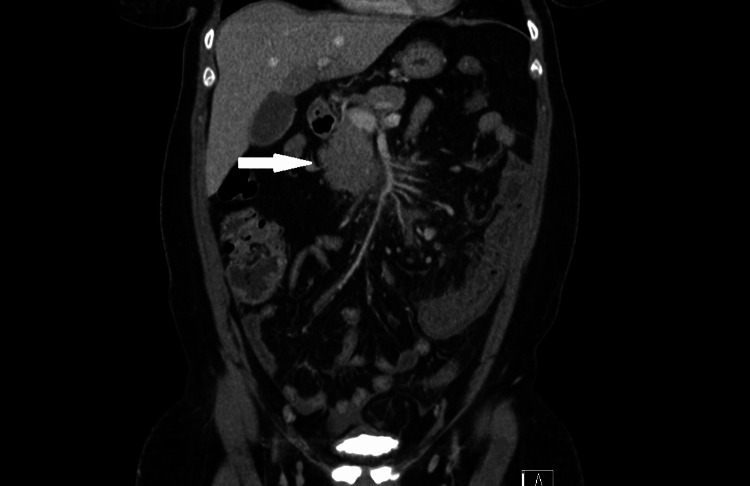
Coronal CT imaging of superior mesenteric vein thrombosis Coronal CT angiography scan highlighting a thrombus in the superior mesenteric vein (arrow)
